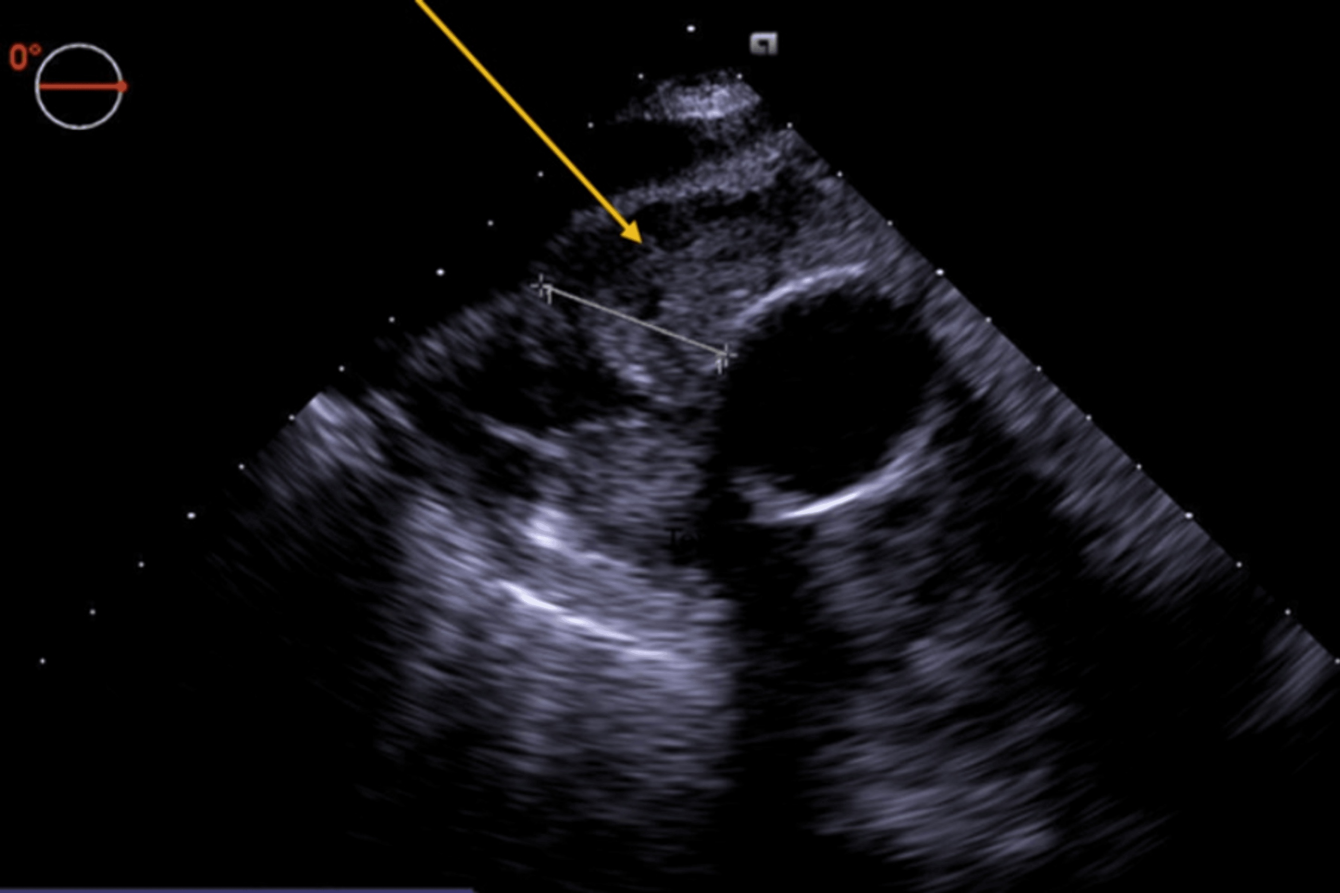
Intraoperative short-axis transesophageal echocardiogram of the mass surrounding the aorta.
